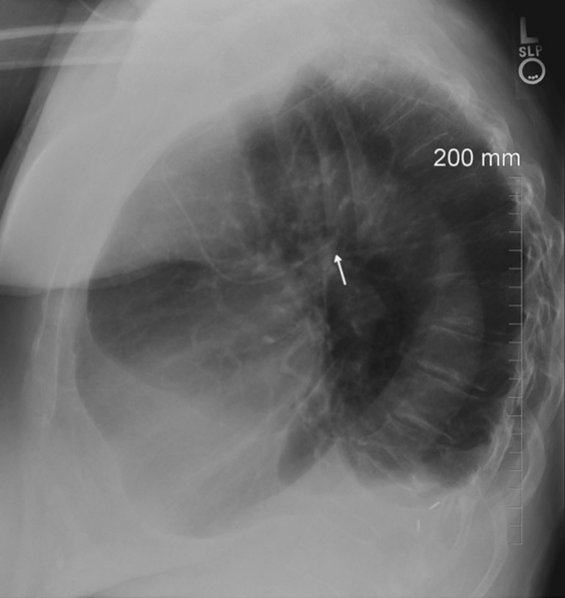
Catheter tip in the azygos vein. Lateral view of the chest X-ray shows the tip of the catheter oriented posteriorly, suggesting dislocation in the azygos vein
